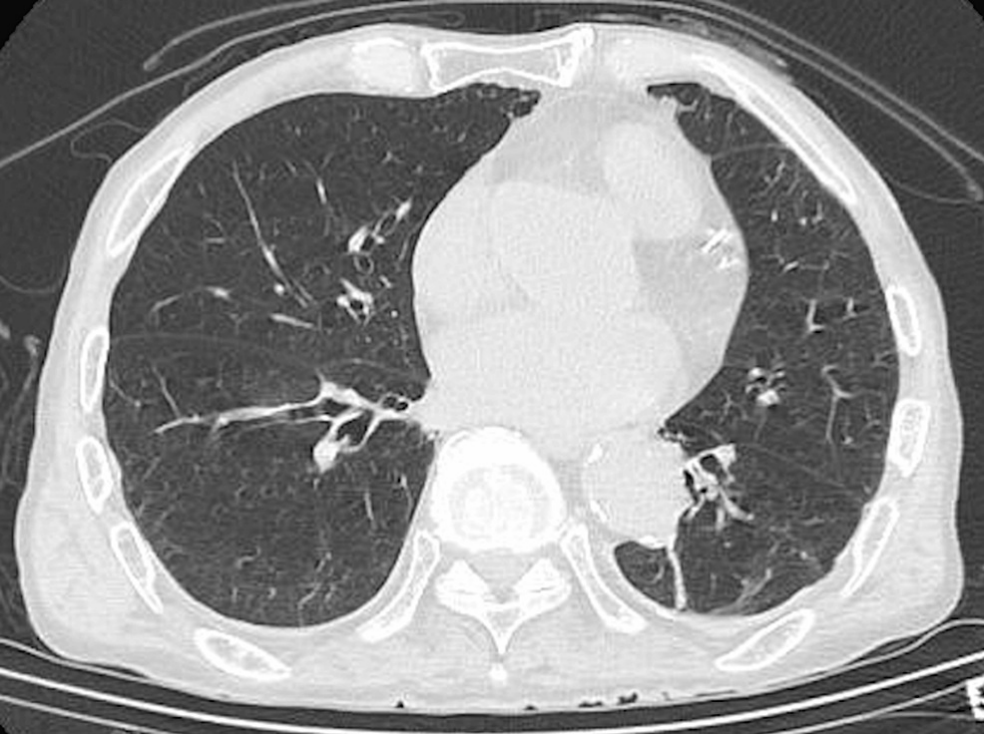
Chest CT which shows no infiltrative shadows
